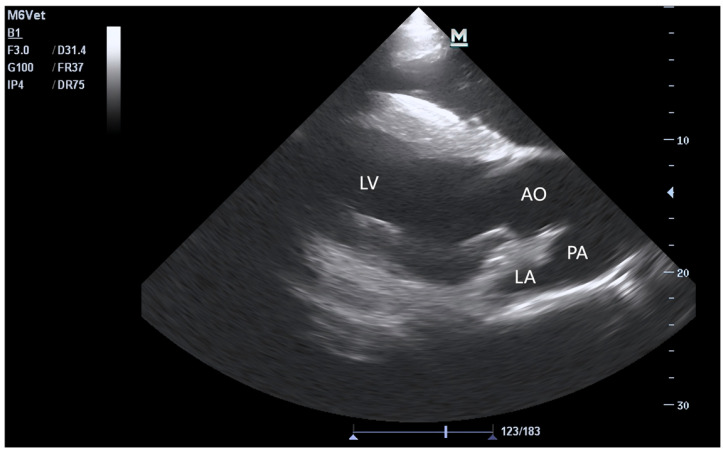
B-mode echocardiogram of the right parasternal long-axis view of the left ventricular outflow tract. PA: pulmonary artery, AO: aorta.
